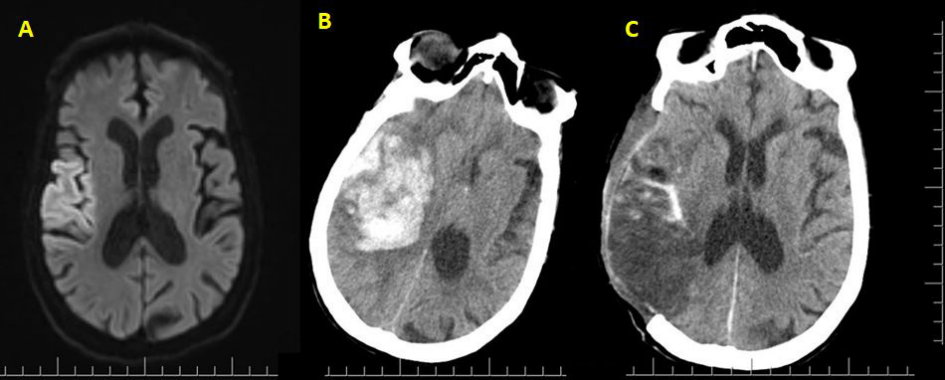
A patient with right MCA infarction. A: Early MRI findings. B: Intracerebral hematoma and subfalcin herniation on CT 24 h after admission. C: CT findings after DHC and hematoma evacuation. *DHC: Decompressive hemicraniectomy, MCA: Middle cerebral artery, CT: Computed tomography, MRI: Magnetic resonance image.
